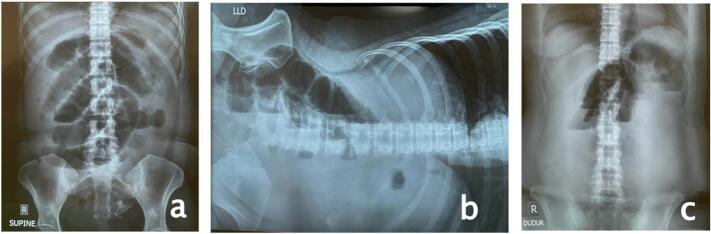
Central dialataion of small intestine on abdominal radiograph a. AP Supine b LLD c. semi erect shows small Bowel obstruction.
